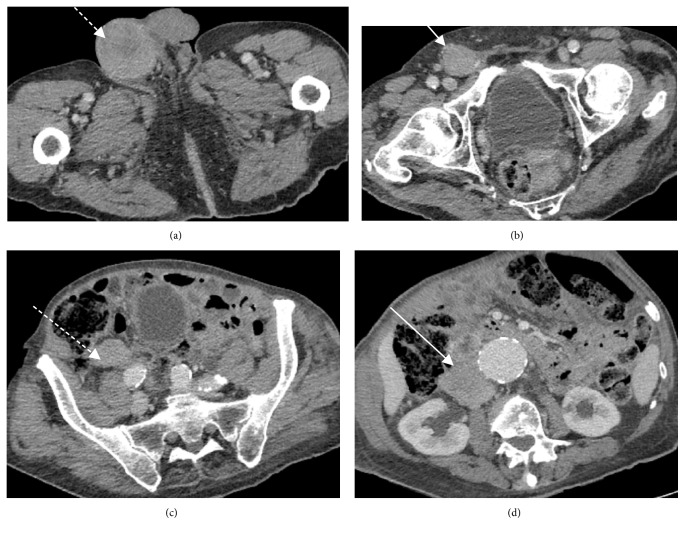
(a) Axial CT image with contrast at the level of the scrotum demonstrates a large, heterogeneous, right testicular tumour (dashed white arrow). (b) Axial CT image with contrast at the level of the right inguinal canal demonstrates extension of homogeneous soft tissue along the right spermatic cord in the inguinal canal (white arrow). (c) Axial CT image with contrast at the level of the common iliac vessels demonstrates tumour anterior to the right iliac artery and vein (dashed white arrow) along the course of the right gonadal vein. (d) Axial CT image with contrast at the level of the right renal pelvis demonstrates soft tissue in the retroperitoneum along the right gonadal vein to the level of its insertion into the inferior vena cava (white arrow).
